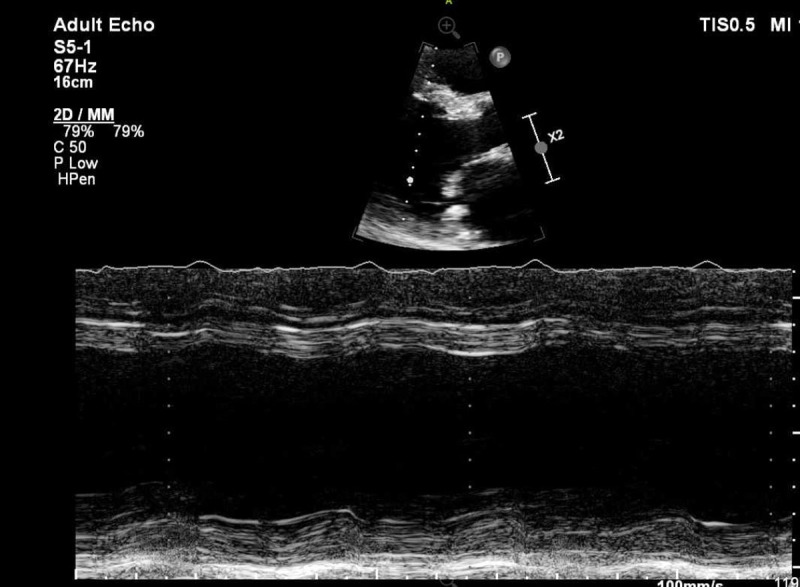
Two-dimensional and M-mode echocardiogram showing a significantly dilated left ventricle.
